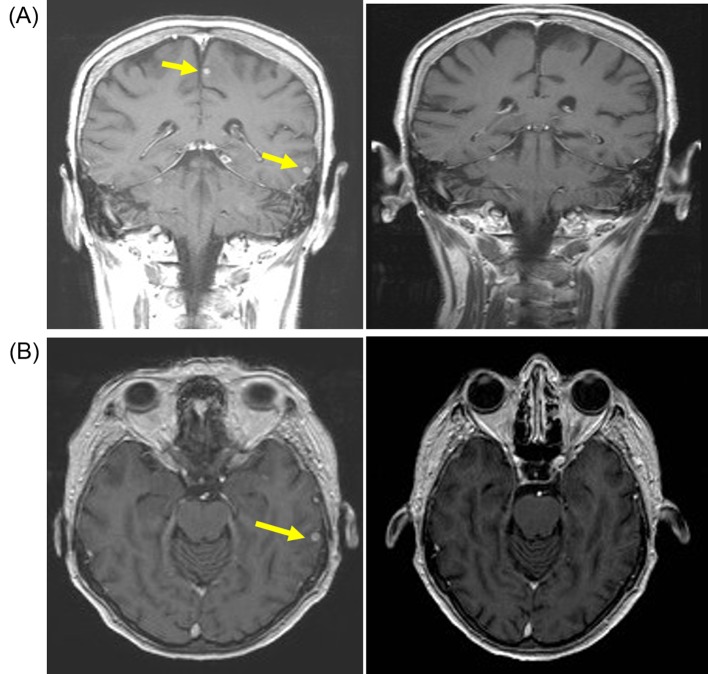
Patient developed response after pazopanib (800 mg/day) with resolution of brain metastasis. (A) Coronal T1-weighted scan with gadolinium contrast demonstrating small sub-centimeter contrast enhancing lesions (yellow arrow) on baseline MRI scan (left panel) that resolved after 3 months of pazopanib (right panel). (B) Axial T1-weighted baseline MRI scan with gadolinium showing small sub-centimeter metastasis (left panel) that resolved after 3 months of pazopanib (right panel).
